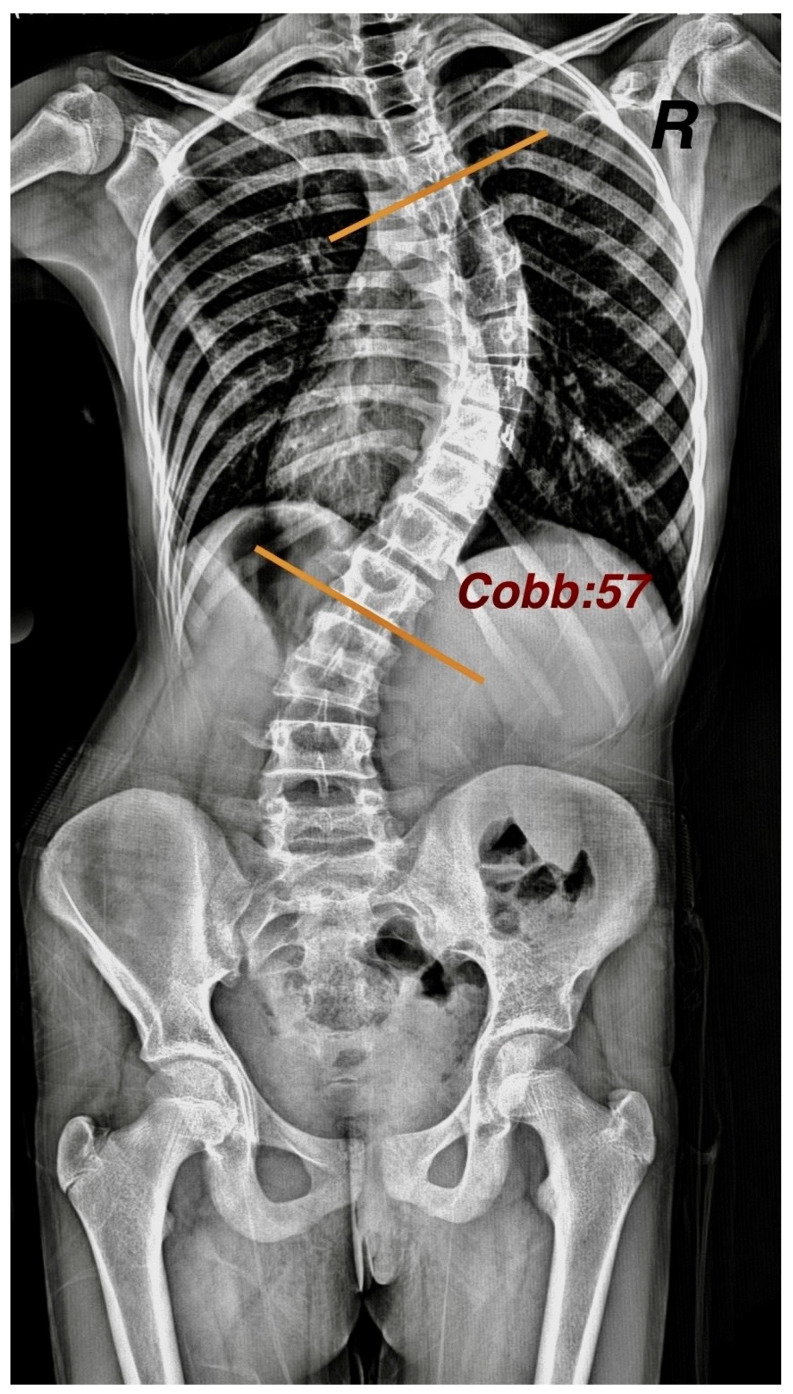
Measurement of Cobb angle on posteroanterior radiograph.
